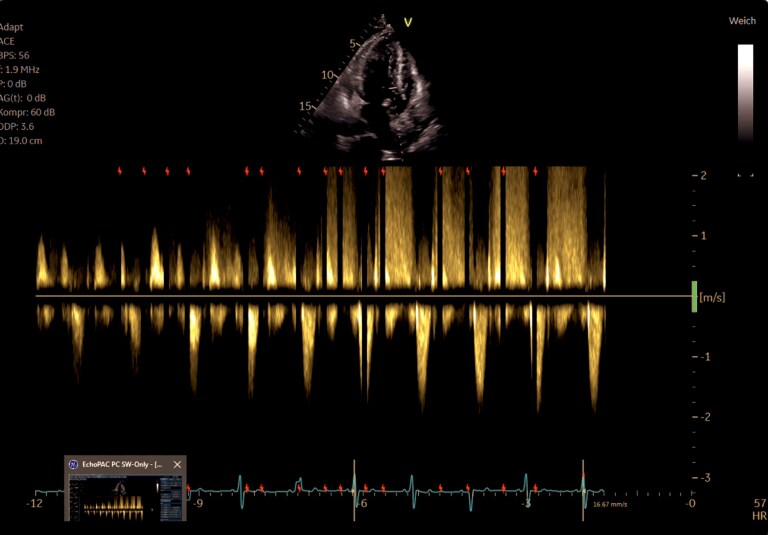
Continuous-wave Doppler across the left ventricular outflow tract postoperatively. Postoperative TTE shows no detectable pressure gradient across the LVOT. Flow velocities remain within physiological range, indicating complete resolution of dynamic or fixed subvalvular obstruction (see Supplementary material online, postoperative TTE  *Videos S1* and *S2*).
